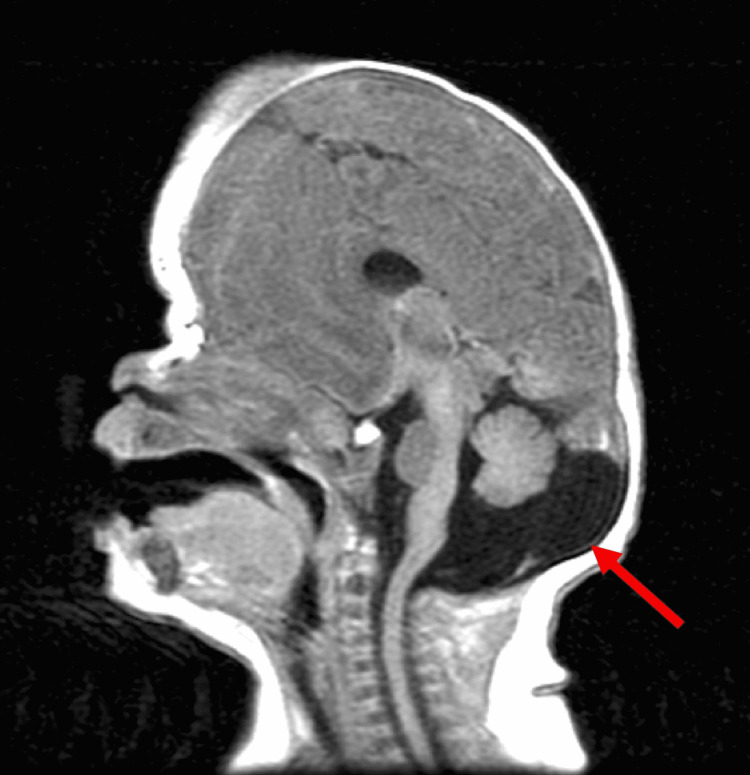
T1-weighted sagittal MRI of the patient. Note the deformation of the skull due to the bilateral coronal synostosis and mega cisterna magna (arrow) MRI: magnetic resonance imaging
